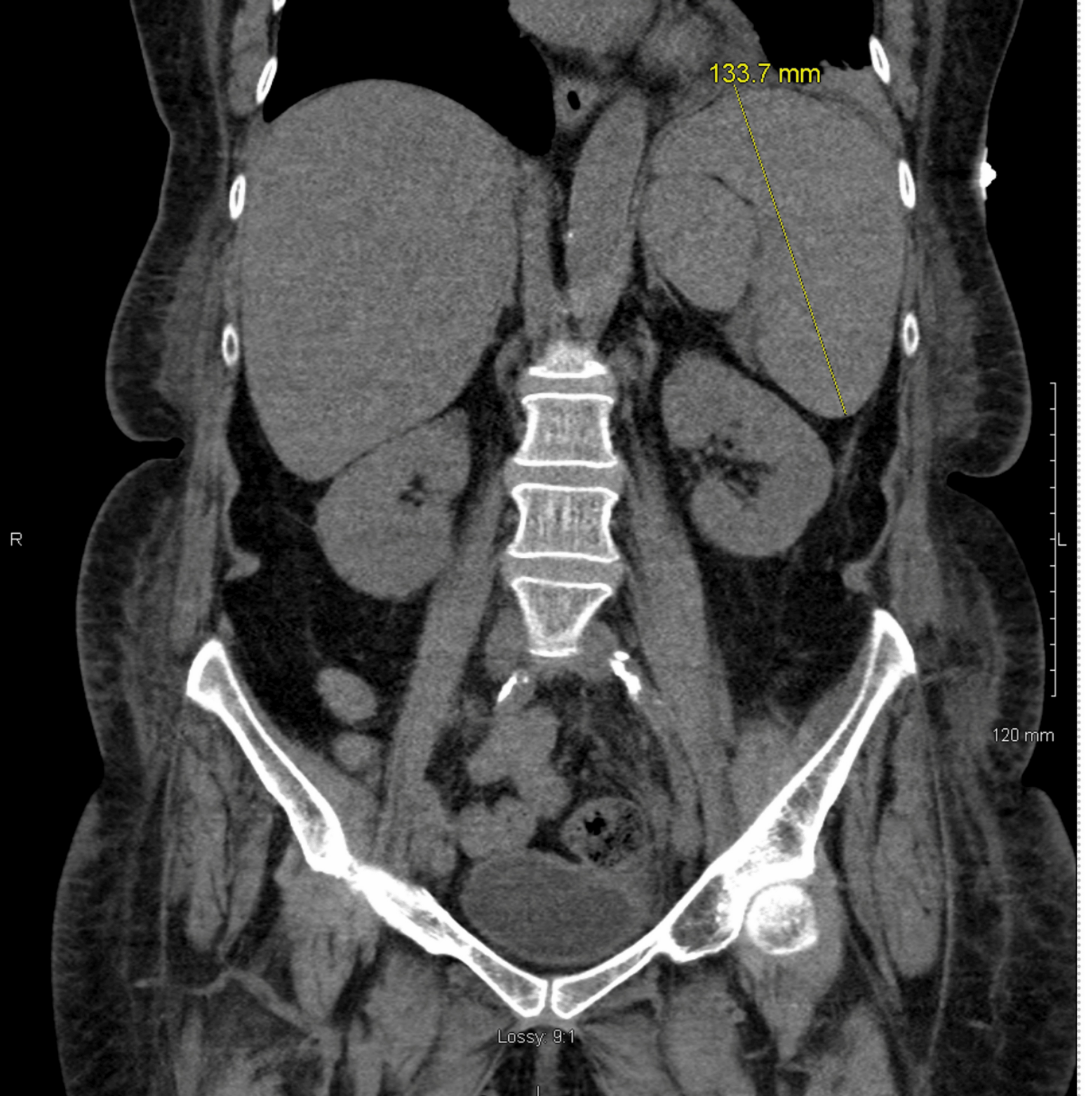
CT of the abdomen and pelvis: splenomegaly up to 13.4 cm (indicated with line). Calculated volume >900 mL
